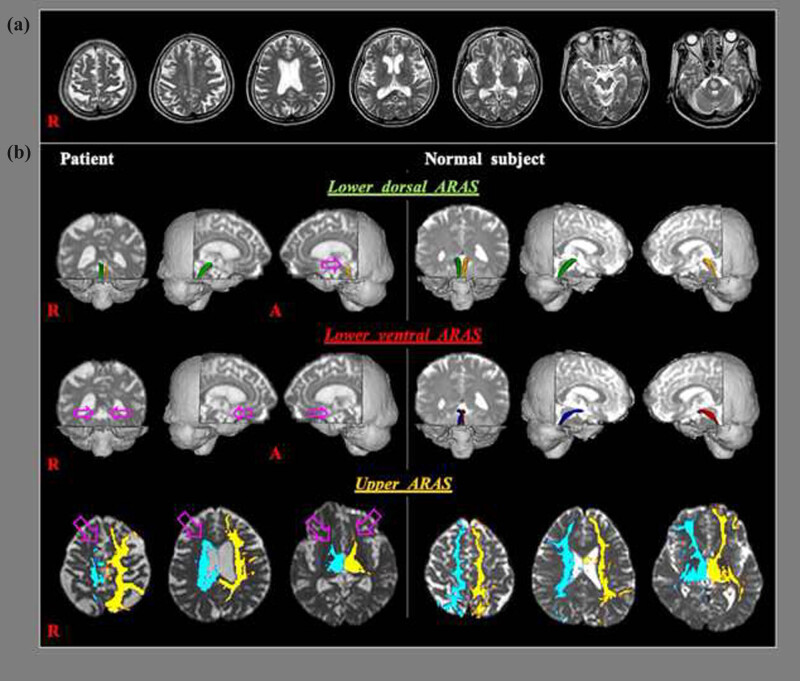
(a) Brain magnetic resonance images taken four weeks after the onset of pontine infarction showing bilateral leukomalatic lesion in the pons. (b) Results of DTT of the ARAS. Impaired neural connectivity (magenta arrows) in the left lower dorsal ARAS (the left lower dorsal ARAS [orange colored tract] is thinner), both lower ventral ARAS (the lower ventral ARAS was not reconstructed in both hemisphere) and both upper ARAS (decreased neural connectivity to each cerebral cortex in both hemispheres: the right hemisphere (sky blue-colored tract)-no neural fibers reached the dorsolateral prefrontal cortex, orbitofrontal cortex, and posterior parietal cortex, and the left hemisphere (yellow-colored tract)-no neural fibers were connected to the orbitofrontal cortex) are noticed compared to a normal subject (73 year-old woman).
